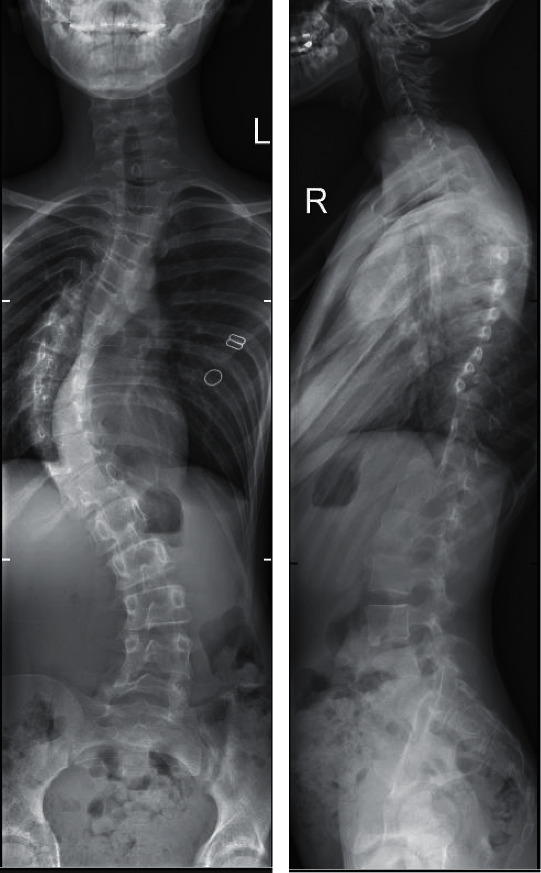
Anteroposterior and lateral X-rays demonstrating right-sided scoliosis.
